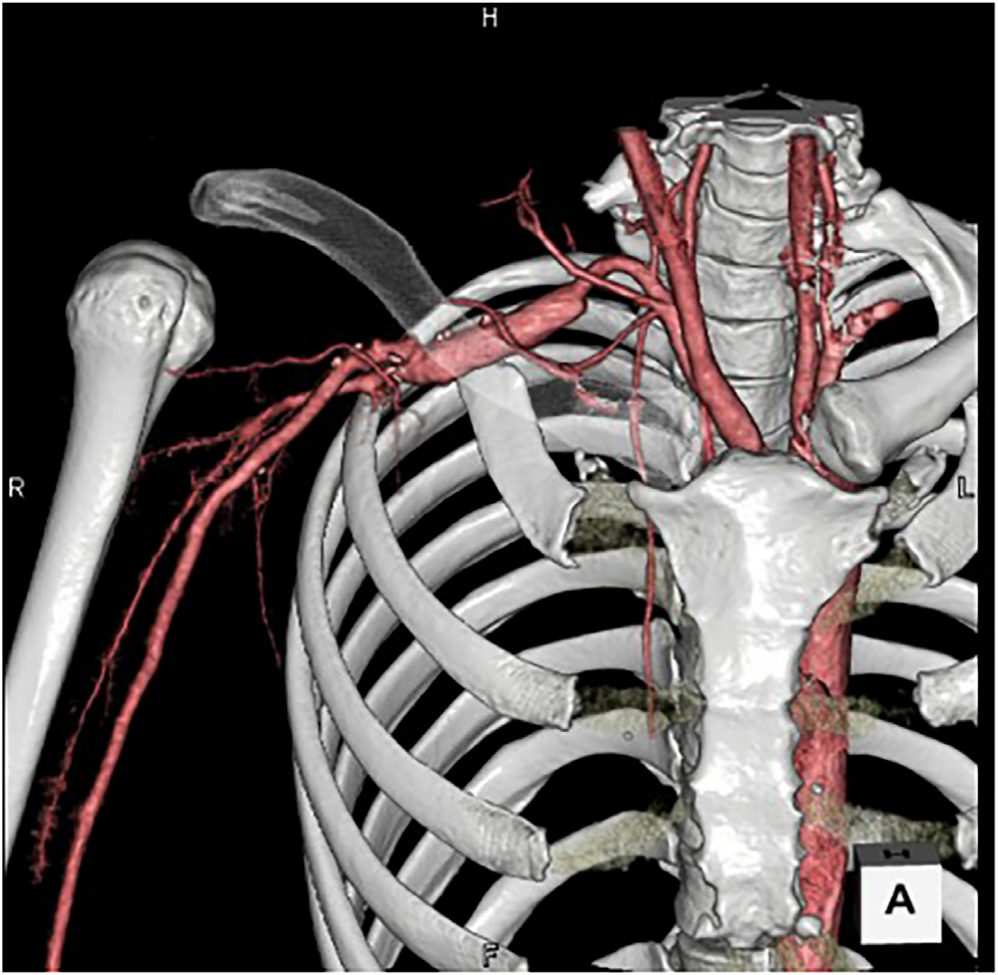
Volume-rendered three-dimensional reconstruction from postoperative contrast-enhanced computed tomography angiography demonstrating restored arterial flow and patency of the reconstructed right subclavian artery following graft interposition.
